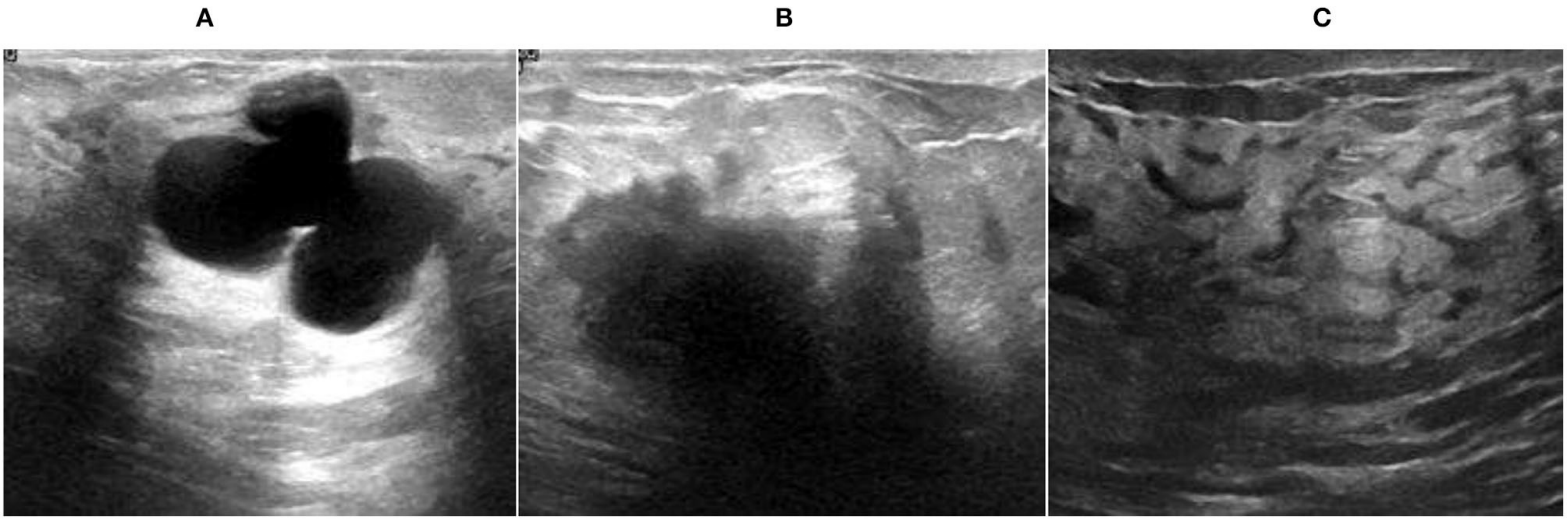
Ultrasound image samples: (A) benign, (B) malignant, and (C) normal.
